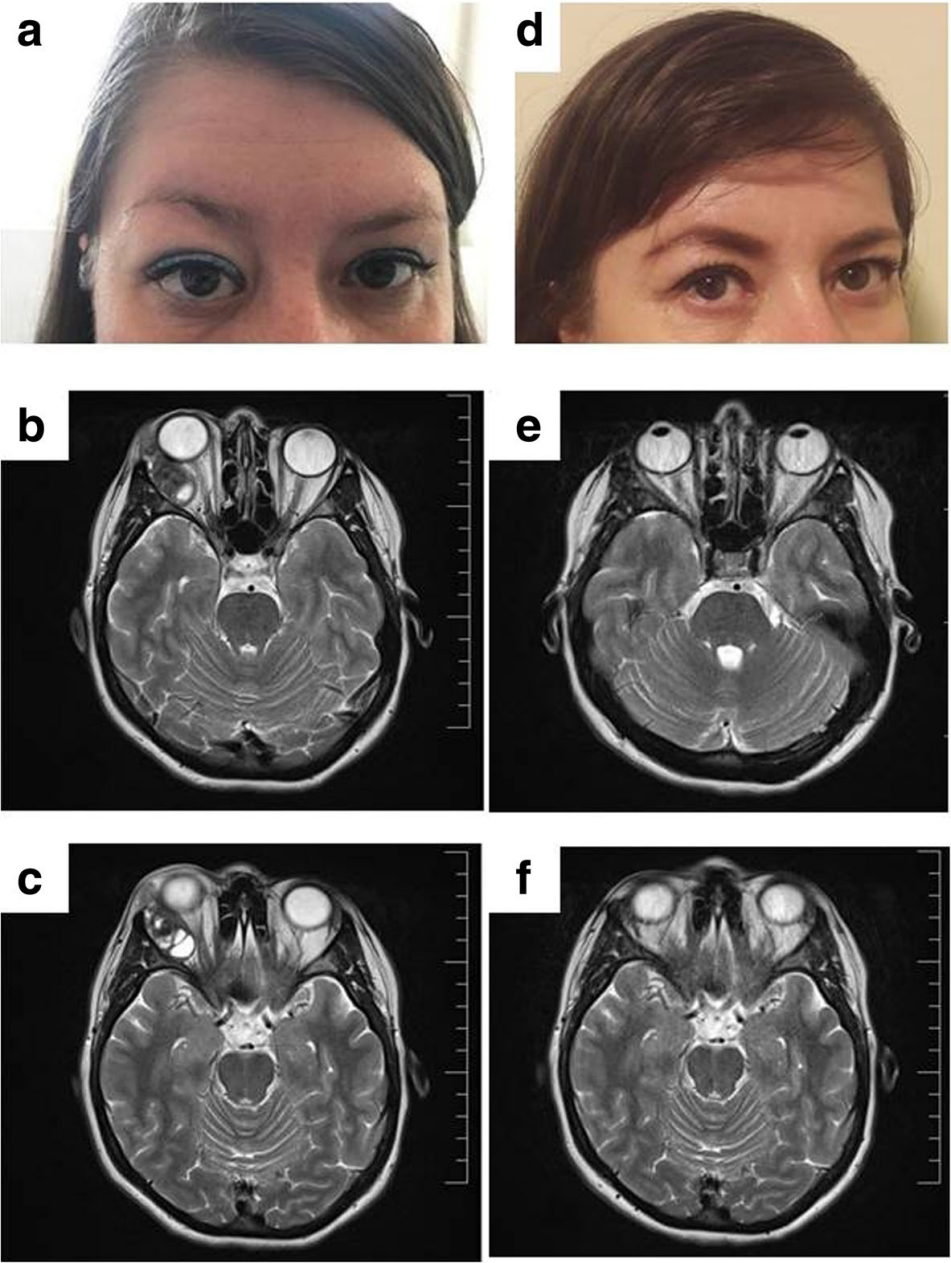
a Preoperative photograph of the patient showing right exophthalmia. b, c MRI detected a well-defined 4 × 2,2 × 2,7 cm mass in the right lacrimal gland region that showed a septate pseudocystic appearance and exerted a mass effect on adjacent structures without local bony destruction. d Postoperative case photograph showing normalization of the globe and resolution of right exophthalmia. e, f No evidence of residual tumour was found in the orbital MRI control
